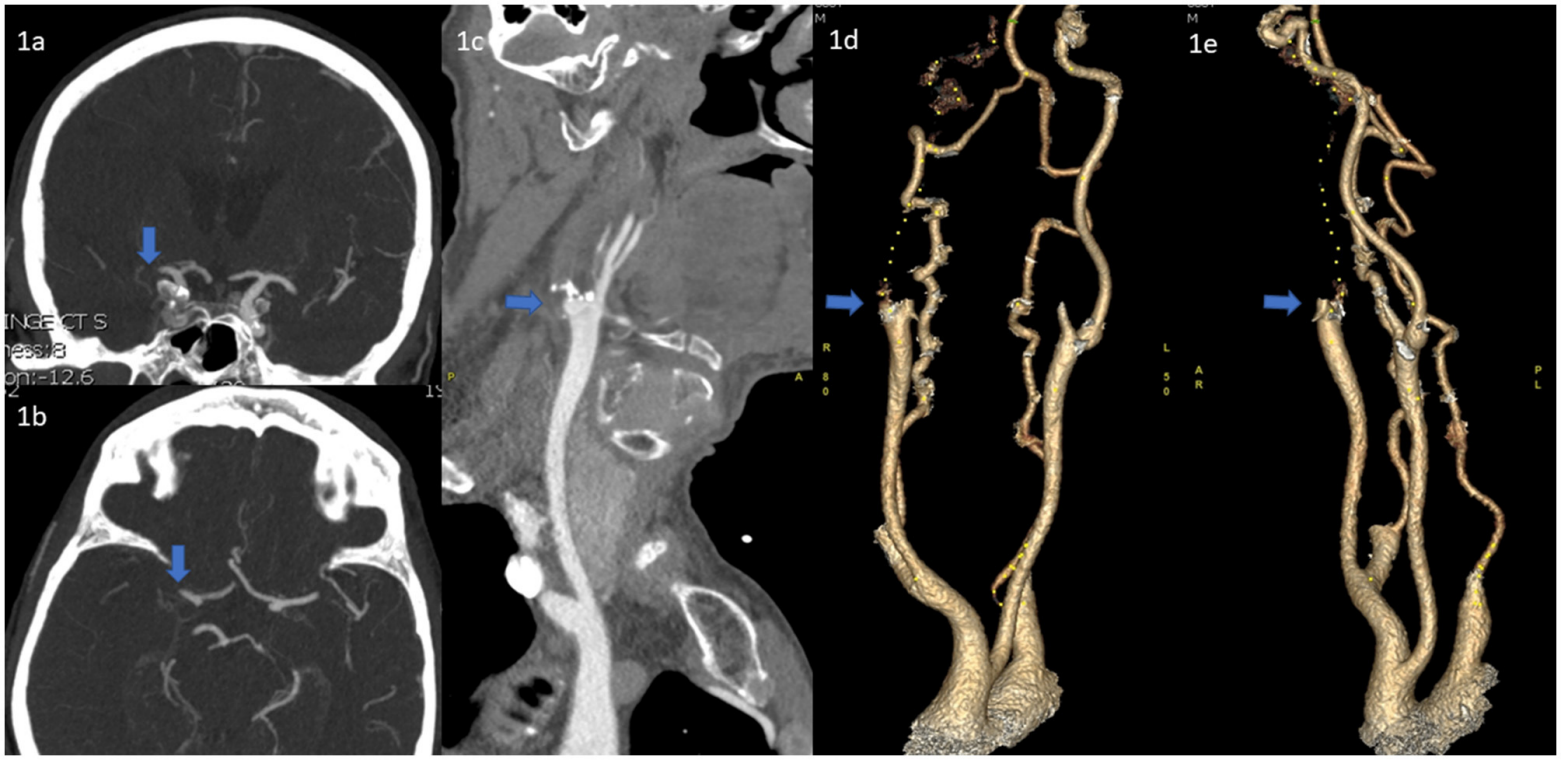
(a, b) CT-A images of the terminal ICA/proximal MCA occlusion. (c) The heavily calcified ICA occlusion at the bifurcation suggestive of an atherosclerotic lesion (d, e) 3d-reconstructions of the same ICA occlusion.
